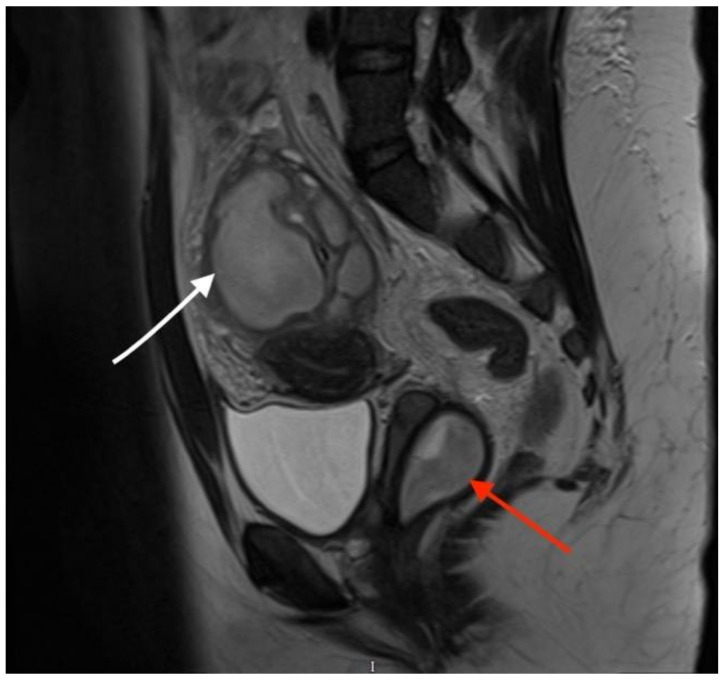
Sagittal T2-weighted MR image showing a vaginal septum with a haematopyocolpos of the obstructed hemivagina and a tubo-ovarian abscess (red arrow indicates the obstructed haematopyocolpos, and white arrow shows the tubo-ovarian abscess). The presence of gas and fluid within the lumen was indicative of a concurrent infection. The patient was initially treated with intravenous antibiotics after the provisional diagnosis of PID. After three days of treatment, she continued to have episodes of pyrexia, the CRP level remained high (242 mg/L) and there were persistently high WCC (16.5 × 10^9^/L) and platelet quantities (500 × 10^9^/L). On the third day after admission, the diagnosis of OHVIRA syndrome was finalised, and the decision was taken to take the patient for surgical intervention to drain the haematocolpos and perform a laparoscopic exploration, to which the patient consented. The next day, the patient underwent an examination under anaesthesia, vaginal septectomy and drainage of the haematopyocolpos. Intraoperative hysteroscopy confirmed the presence of a uterus didelphys bicollis. The cervix of the affected cavity was dilated to encourage continuous drainage. Subsequently, during the laparoscopy, significant pelvic adhesions were noted, involving the bowel being adherent to the uterus, and a large right-sided tubo-ovarian abscess. Laparoscopic adhesiolysis was performed, as well as the drainage of the abscess. The pelvic drain was left in situ at the end of the procedure. She remained an inpatient for four days after surgery. The drain was removed three days after the operation. She was continued on intravenous antibiotics for a further three days before they were converted to oral medication. She no longer had a post-operative spiking temperature, and her pulse normalised two days after the operation. Her CRP dropped to 51 mg/L and WCC to 9.5 × 10^9^/L on the day of discharge. She was followed up in the Gynaecology Clinic with repeat imaging that confirmed the resolution of the tubo-ovarian abscess. She was carefully debriefed about the implications of having uterus didelphys and a single kidney in pregnancy and was further signposted to written information provided by the British Society of Paediatric and Adolescent Gynaecology.
